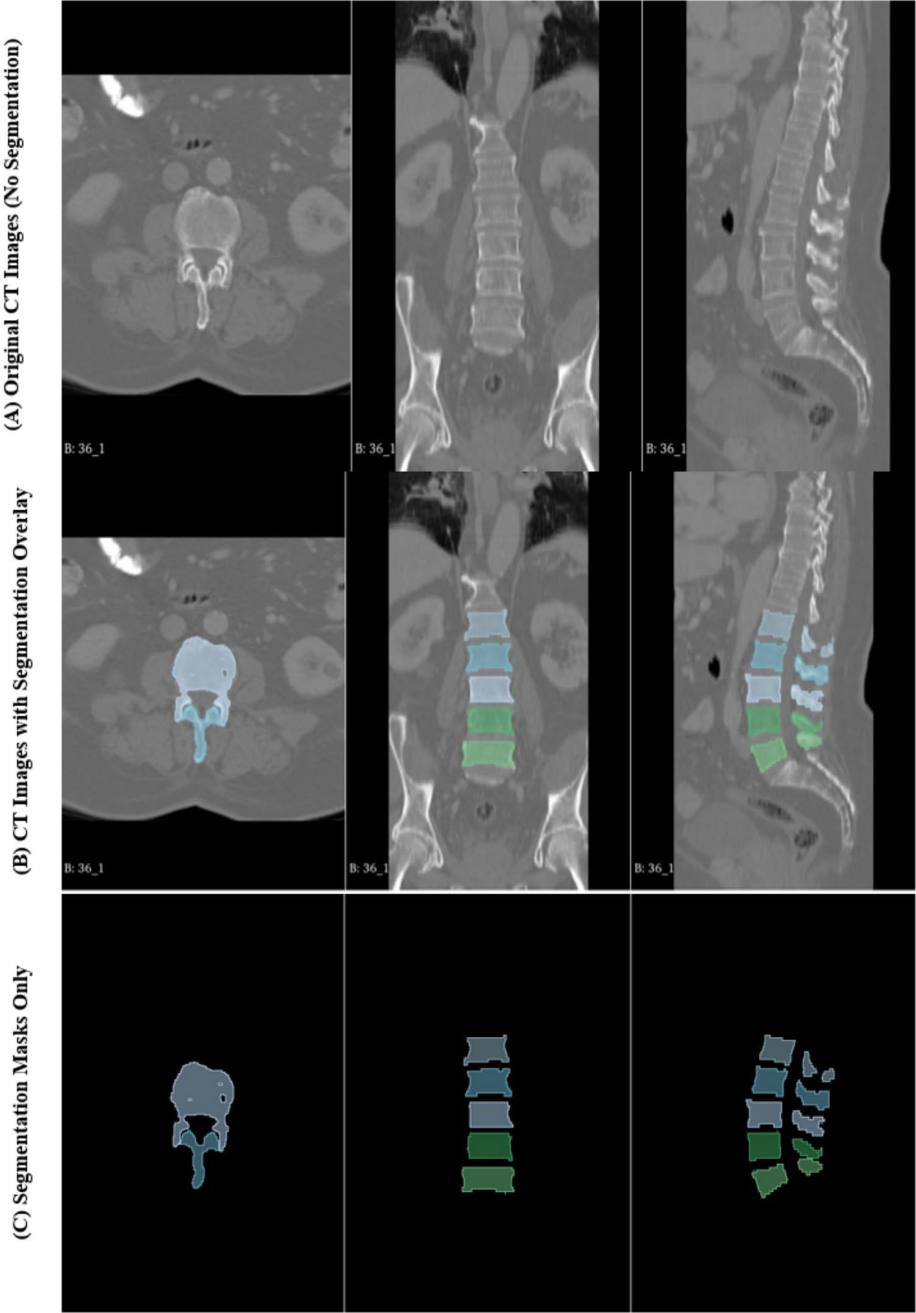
AI-assisted vertebral segmentation from CT imaging
